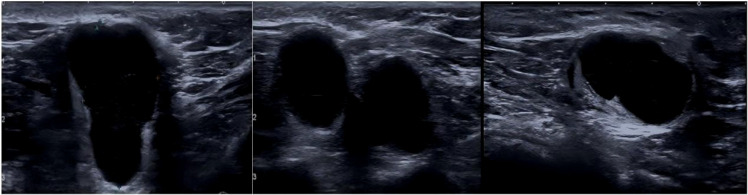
Axillary lymph nodes (LNs) show suspicious features at ultrasound (US), including cortical thickening more than 3 mm, round or irregular shape, and loss of fatty hilum at presentation.
